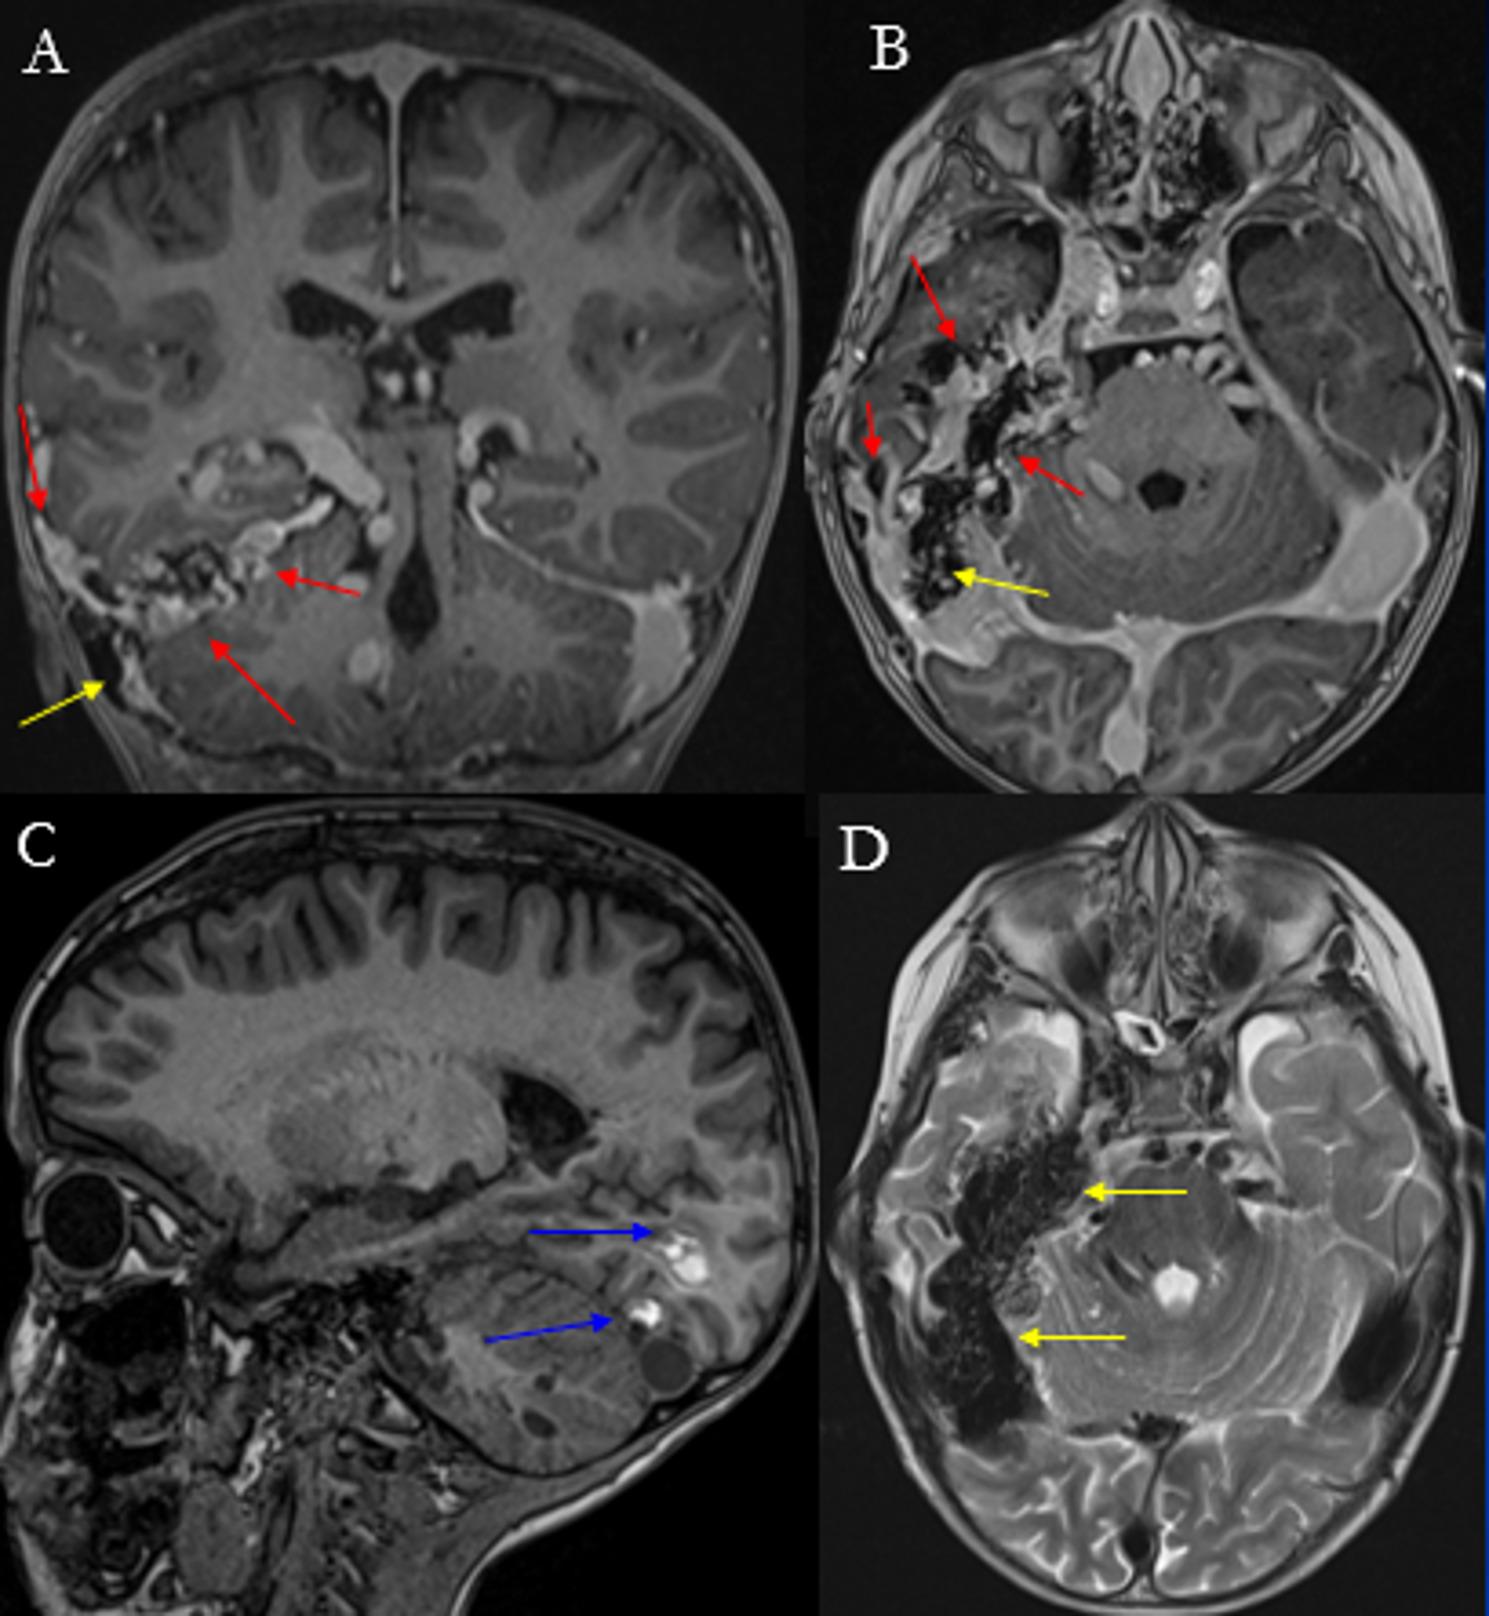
Child with PTEN hamartoma tumor syndrome/Cowden syndrome. (A and B) Post-contrast coronal and axial T1-weighted image (WI), (C) pre-contrast sagittal T1WI, and (D) axial T2WI. There are many small dural fistulas (in the floor of the right middle cranial fossa, posterior fossa and along the tentorial leaflet with curvilinear vascular enhancement due combination of feeding arterial branches from posterior cerebral artery and inferior middle cerebral artery division branches and draining veins (red arrows in A and B) in conjunction with dilated dural sinuses. Post embolization changes with hypointense coiling materials are also noted (yellow arrows in A, B and C). Additionally, two small cavernomas with hyperintense T1 subacute hemorrhage are present in the occipital lobe on the right (blue arrows in C)
